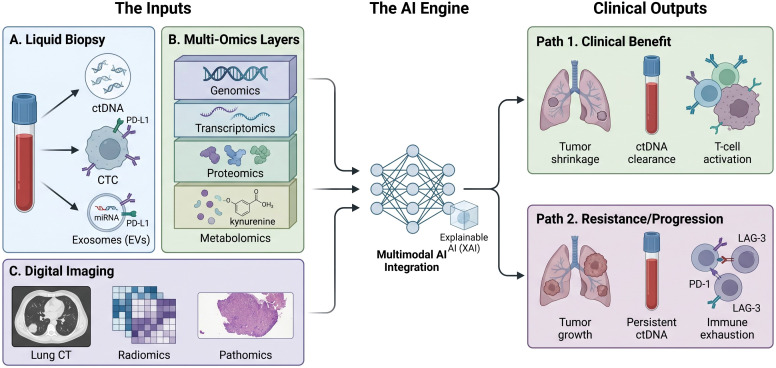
Dynamic, multi-parametric framework for precision immuno-oncology. The Inputs: The framework integrates non-invasive data from three distinct dimensions: (A) Liquid Biopsy, capturing circulating tumor DNA (ctDNA), CTCs, and extracellular vesicles (EVs) carrying cargo such as PD-L1 and miRNA; (B) Multi-Omics Layers, encompassing genomics, transcriptomics, proteomics, and metabolomics (e.g., kynurenine levels); and (C) Digital Imaging, including lung CT scans and derived radiomics and pathomics features. The AI Engine: Multimodal AI integration is utilized for non-linear pattern recognition across disparate data types. Clinical Outputs: Patients are stratified into two primary trajectories: Path 1 (Clinical Benefit), characterized by tumor shrinkage, ctDNA clearance, and robust T-cell activation; and Path 2 (Resistance/Progression), marked by tumor growth, persistent ctDNA, and immune exhaustion, often identified by the co-expression of multiple checkpoints.
